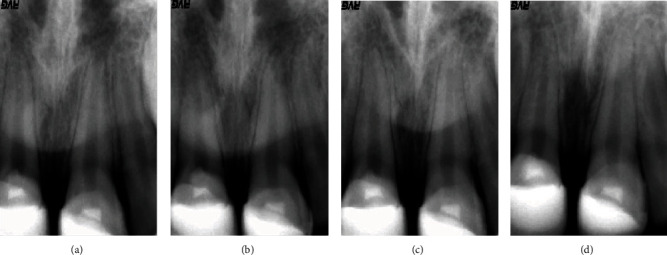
Periapical radiography, at 2 months (a), 1 year (b), 2 years (c), and 3 years (d) after trauma.
